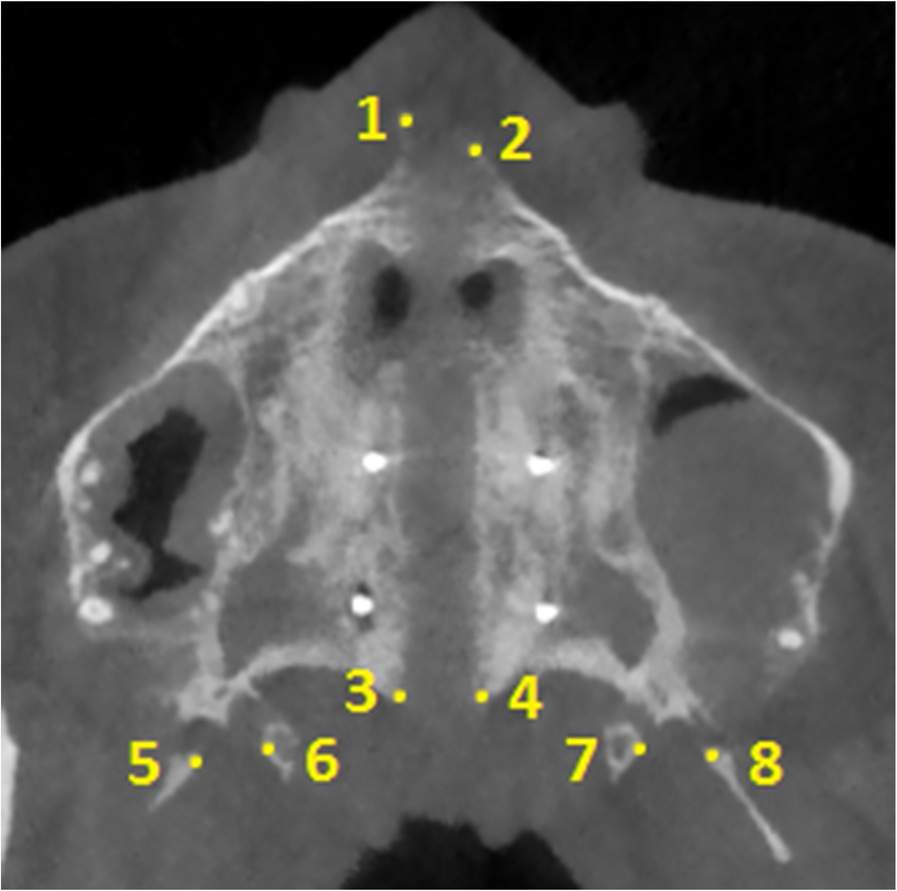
Landmarks identified in the axial palatal section in the post-expansion CBCT. 1 Right anterior nasal spine (Rt ANS), 2 left anterior nasal spine (Lt ANS), 3 right posterior nasal spine (Rt PNS), 4 left posterior nasal spine (Lt PNS), 5 most medial point of the lateral plate of the right pterygoid process (Rt Lat Pter), 6 most lateral point of the medial plate of the right pterygoid process (Rt Med Pter), 7 most lateral point of the medial plate of the left pterygoid process (Lt Med Pter), 8 most medial point of the lateral plate of the left pterygoid process (Lt Lat Pter)
